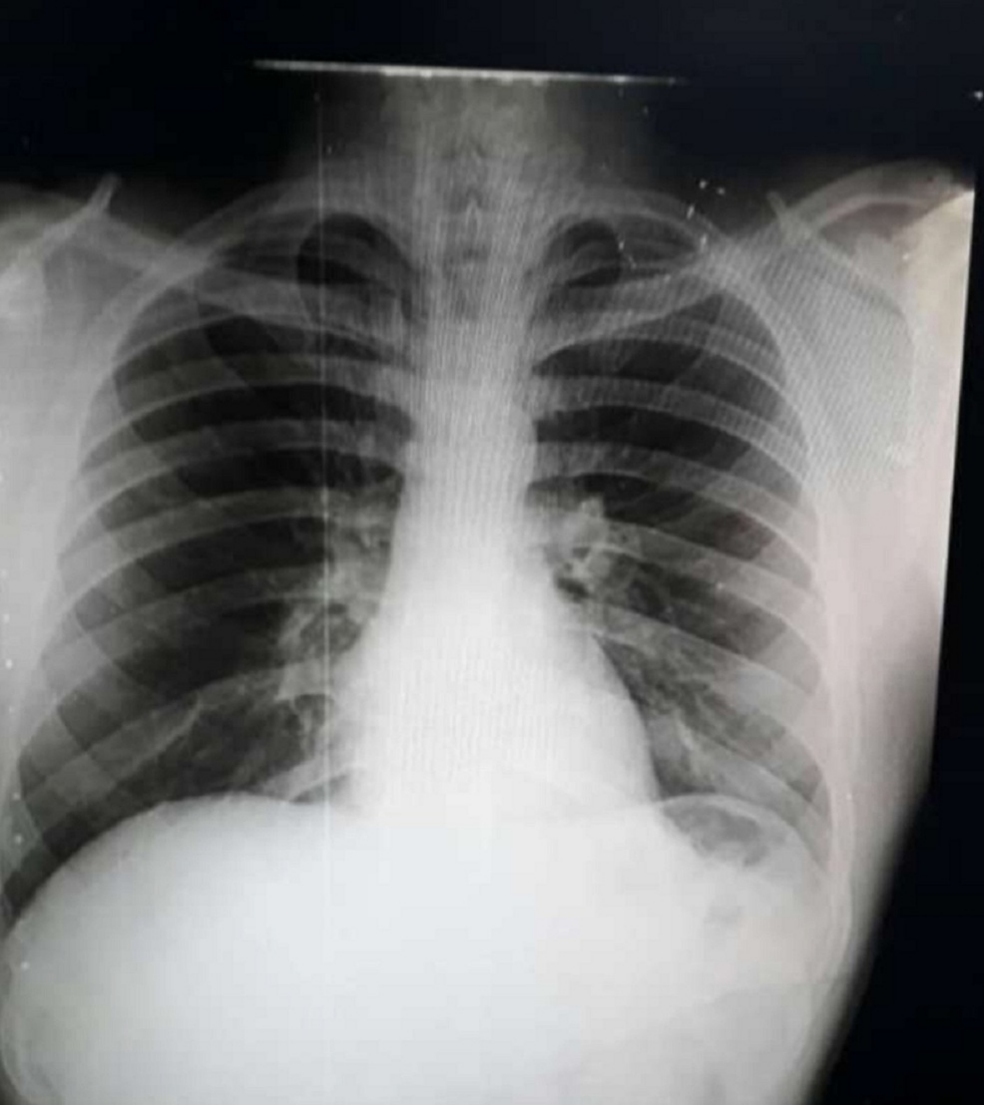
Digital radiograph of the chest showing successful maintenance of reduction of the left sternoclavicular joint.
